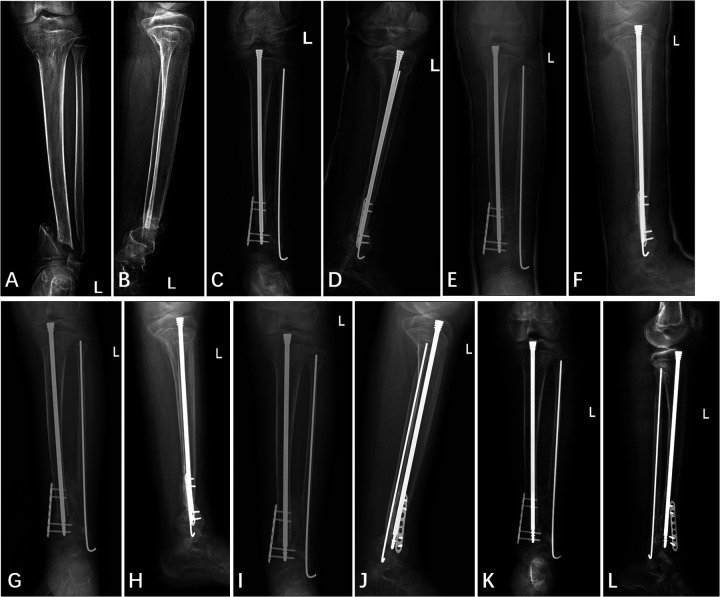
Anterior and lateral x-ray film of left tibia and fibula (A,B) one day pre-operation, (C,D) one day post-operation, (E,F) two months post-operation, (G,H) four months post-operation, (I,J) five months post-operation, and (K,L) twelve months post-operation.
